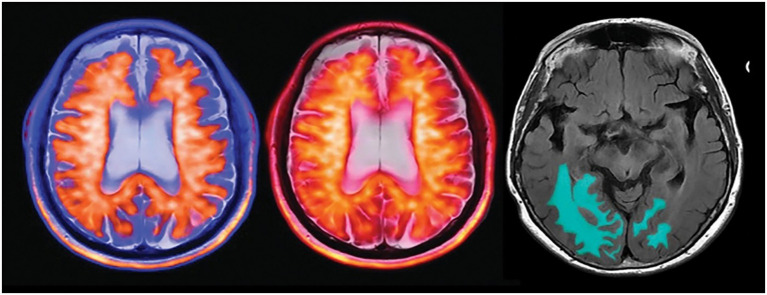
Multimodal imaging and AI-assisted detection of early Alzheimer’s disease using PET-MR fusion and automated MRI analysis.
